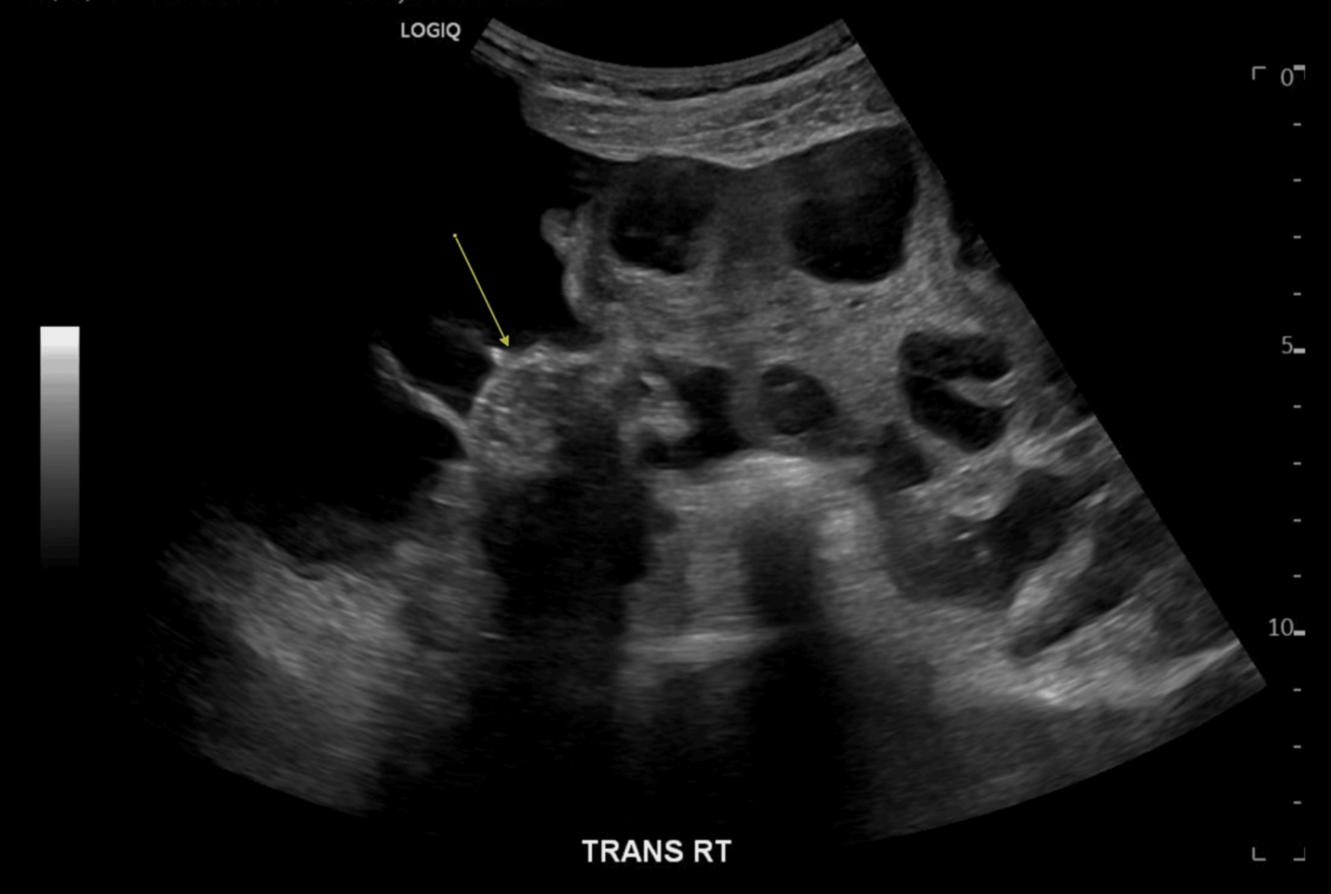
Transvaginal and transabdominal ultrasound Revealing marked ovarian enlargement (arrow) with hemorrhagic theca lutein cysts, moderate free fluid, and mild swirling of the right infundibulopelvic ligament.
